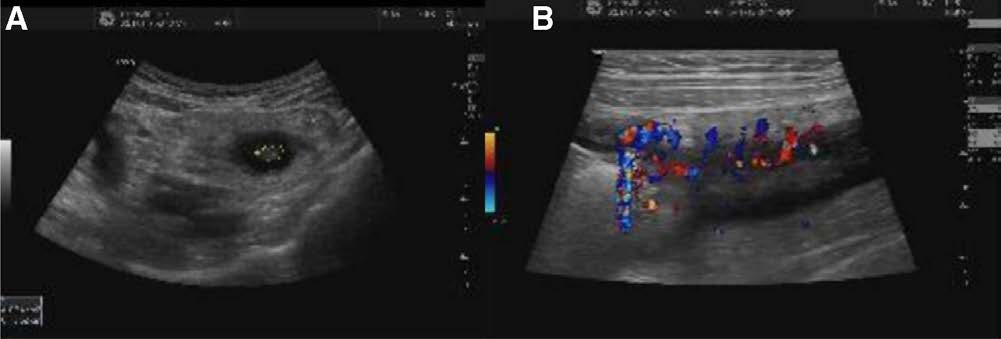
Intestinal wall thickening, echo reduction in the descending and the sigmoid colon was observed, the intestinal lumen was significantly narrowed (A), and the blood flow signal increased (B) as seen in the transabdominal ultrasound.
